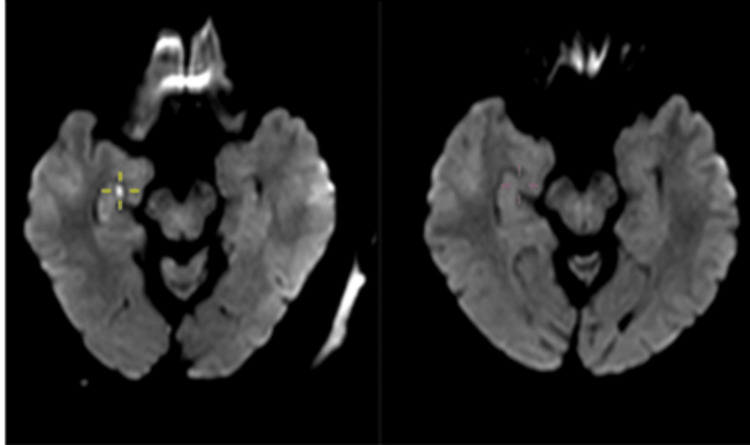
Case 2: Image on the left side shows a small area of restricted diffusion (cross-marked) in the right hippocampal head. Image on the right side shows complete resolution of the area of restricted diffusion on a follow-up MRI.
